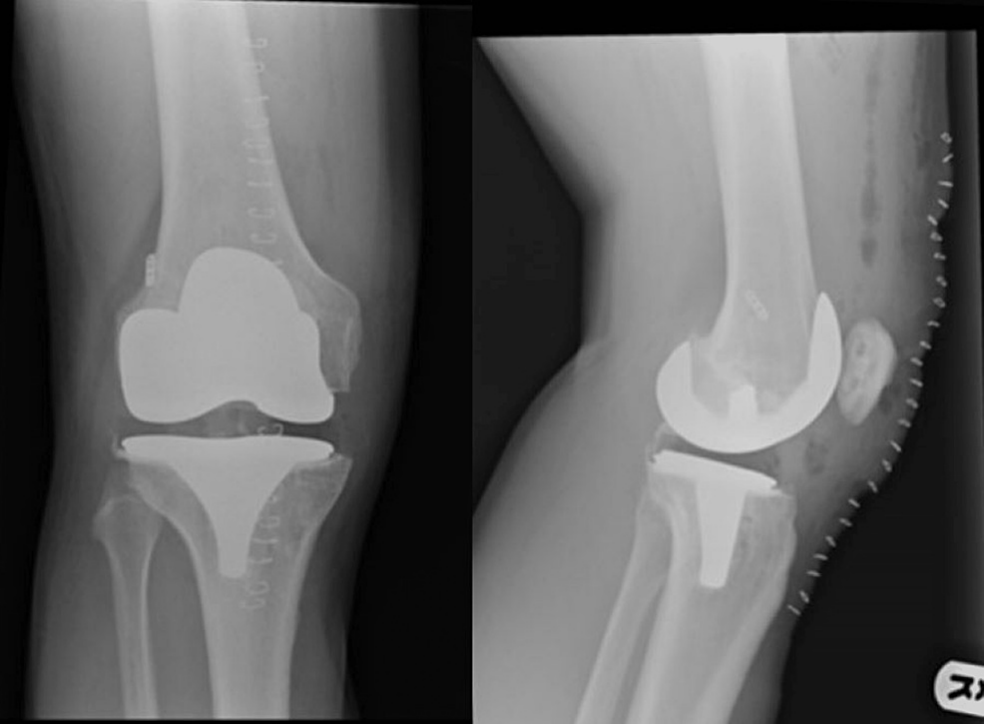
Post-operative radiographs done in February 2016. Antero-posterior (left) and lateral (right) radiographs following primary knee arthroplasty.
